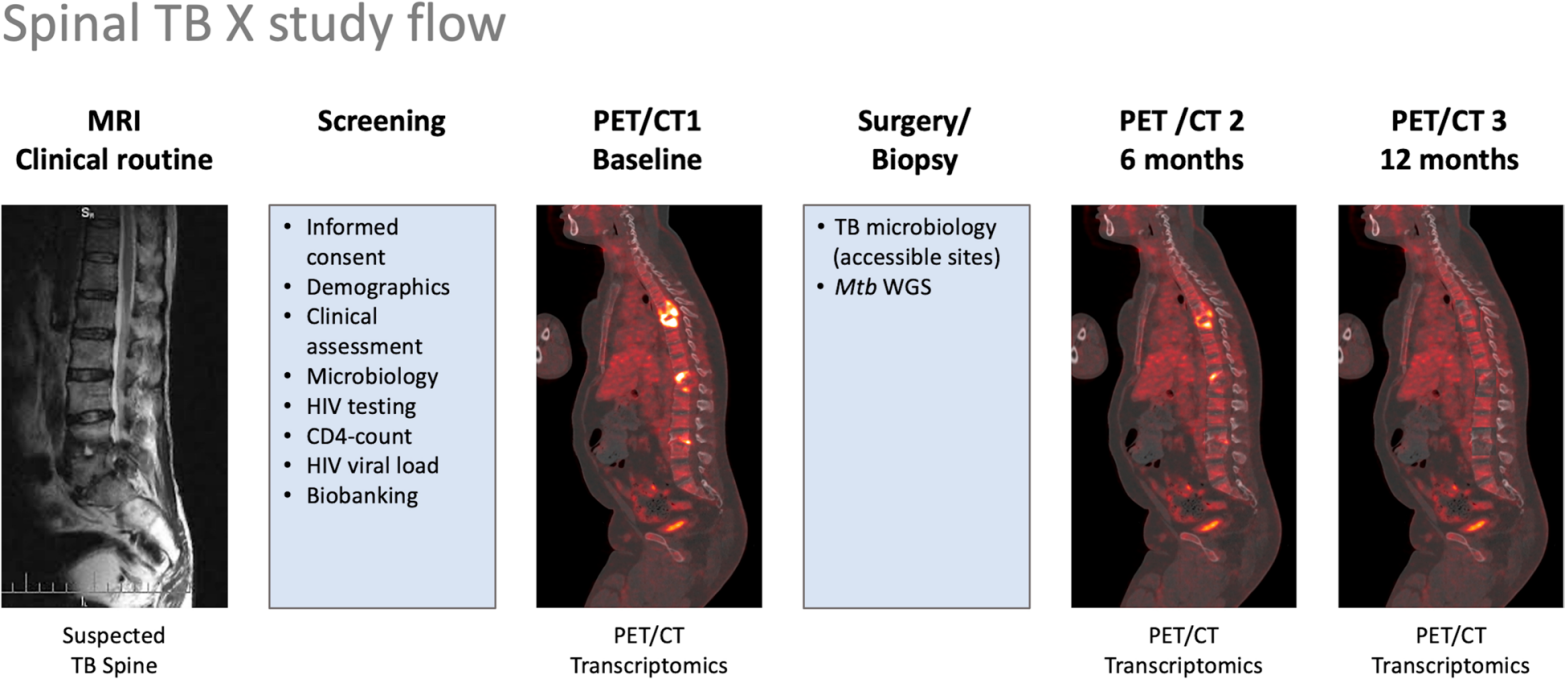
Spinal TB X study flow. *TB* tuberculosis, *MRI* magnetic resonance imaging, *PET*/*CT*
